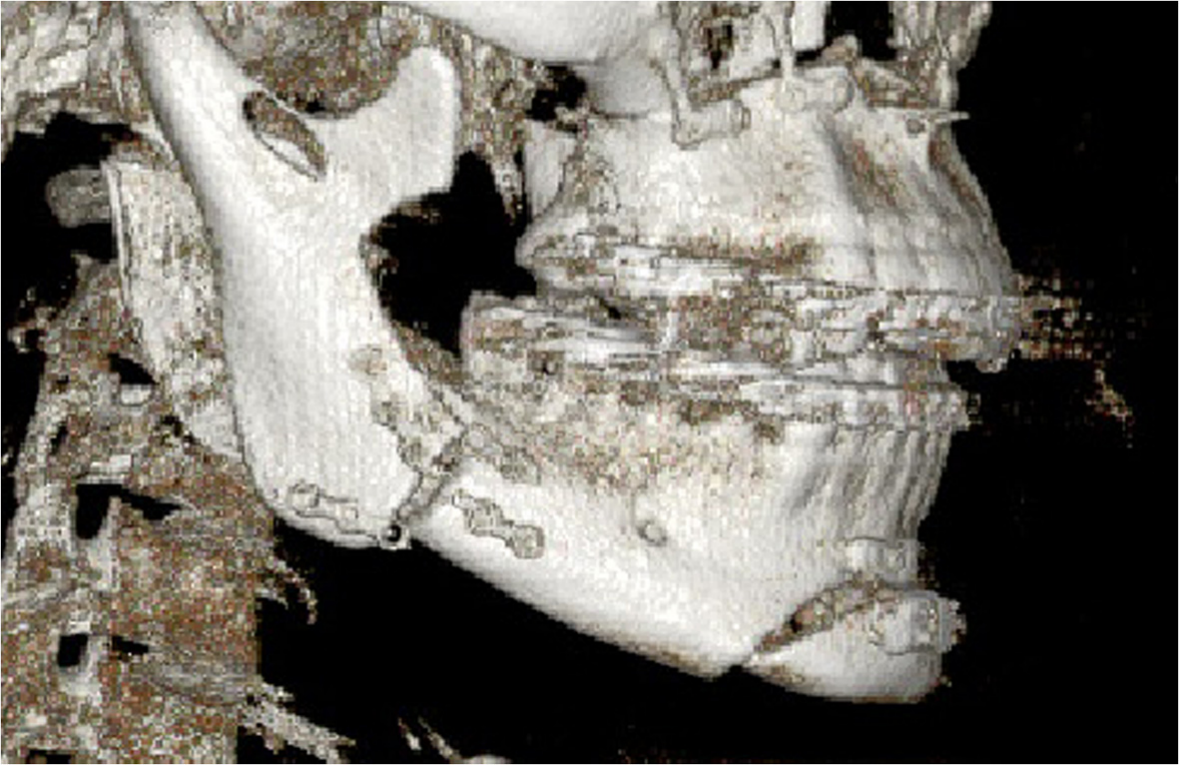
3-dimensional reconstructed CT image showing that two mini-sized, bioabsorbable plates are fractured 7 weeks after implatation.
